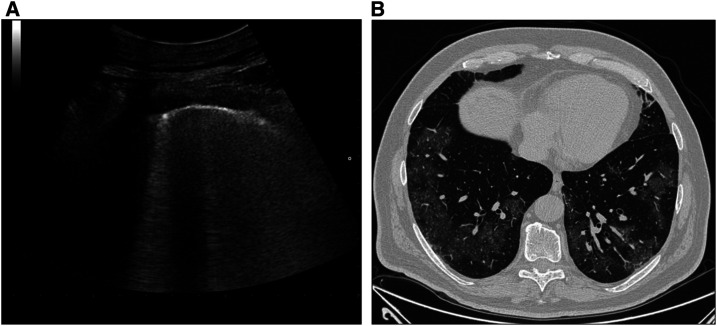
(A) Ultrasound scan of the right lung parenchyma using the convex transducer that shows B-lines. (B) Axial high-resolution computed tomography image of the same region showing bilateral and diffuse ground-glass opacities.
